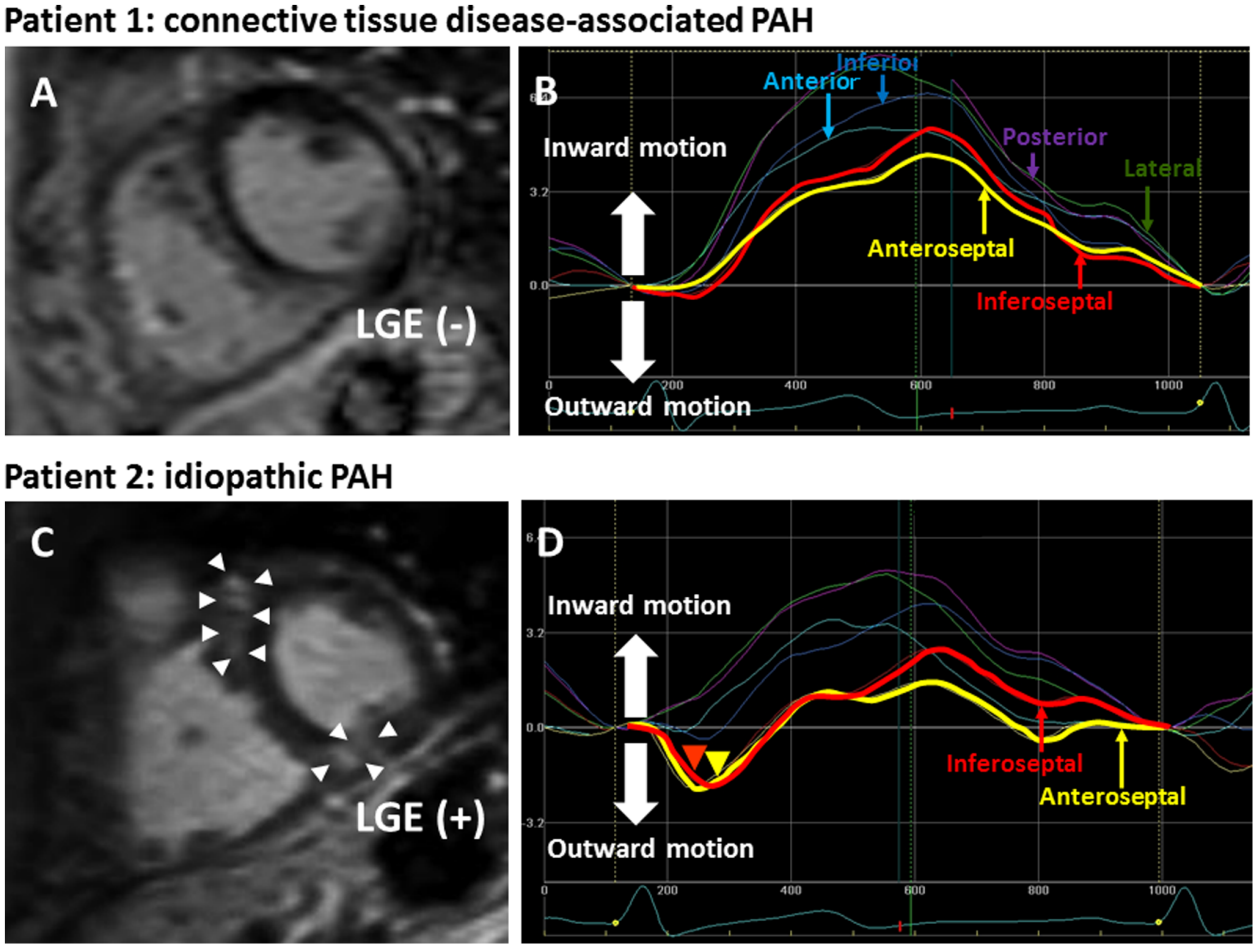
Representative images of cardiac magnetic resonance and speckle tracking echocardiography with or without late gadolinium enhancement and paradoxical motion of the interventricular septum. Patient 1 had connective tissue disease-associated PAH with a mean pulmonary artery pressure of 43 mmHg. CMR (A) shows no late gadolinium enhancement at ventricular insertion points. There is no paradoxical motion of the interventricular septum by speckle tracking echocardiography (B). Patient 2 had idiopathic PAH with a mean pulmonary artery pressure of 37 mmHg. Late gadolinium enhancement at ventricular insertion points is shown in a CMR image (arrow heads, C), and paradoxical motion of the interventricular septum at early systolic phase (arrow heads) is also noted on speckle tracking echocardiography (D). PAH, pulmonary artery hypertension; CMR, Cardiac magnetic resonance.
